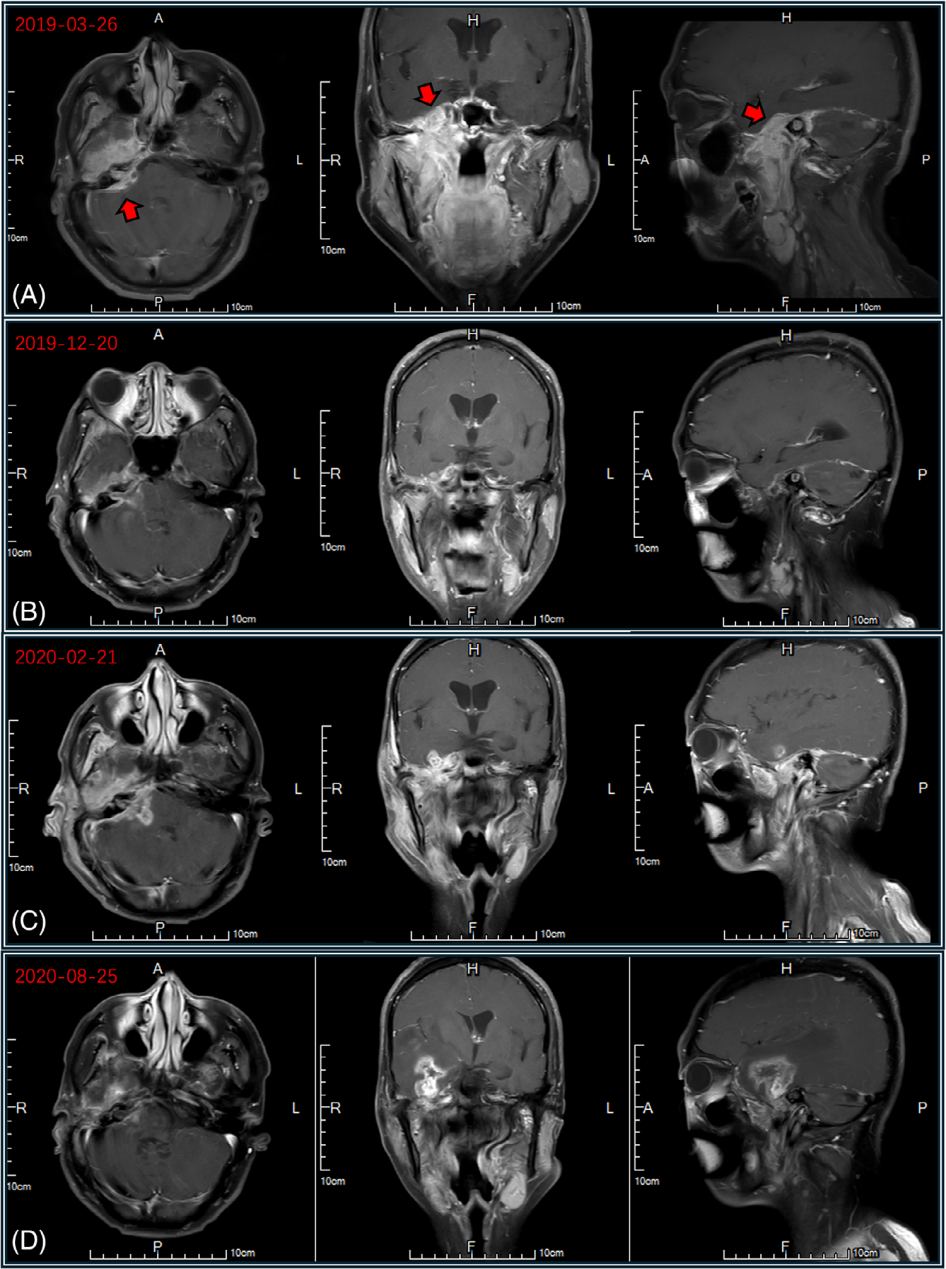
Changes in the tumor observed on magnetic resonance imaging scans at different time points. A) Before treatment. B) After the initial treatment. C) Progression after treatment. D) After carboplatin and 5‐fluorouracil chemotherapy combined with pembrolizumab.
